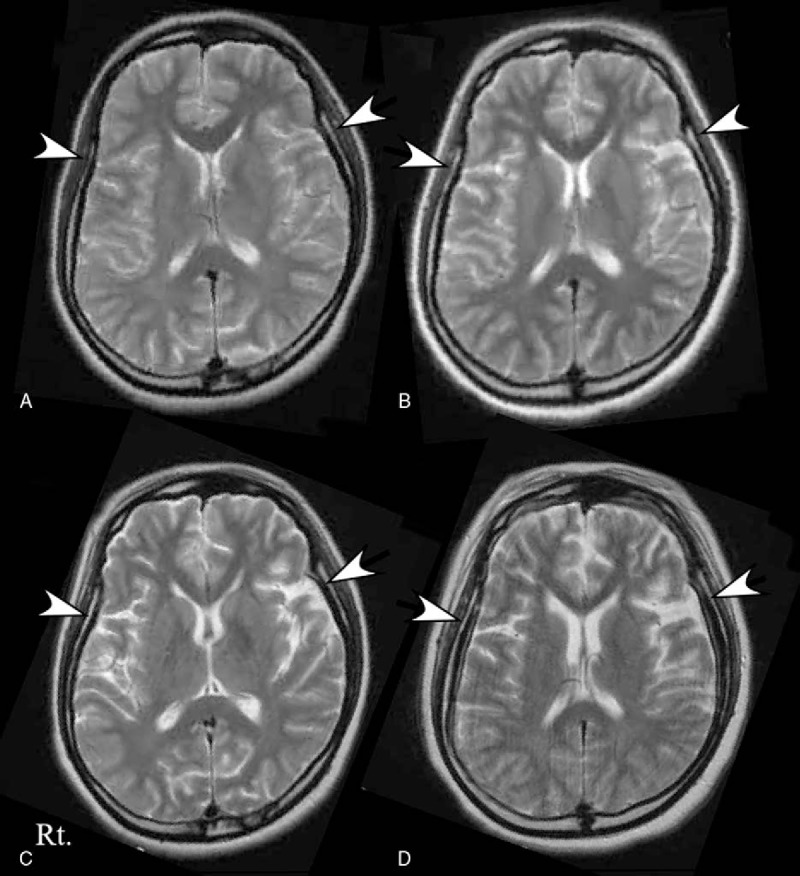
Serial MRI studies in Patient 2. The first brain MRI was normal (panel A). The second brain MRI, obtained on day 19, showed the presence of diffuse brain atrophy (panel B, white arrows). The diffuse brain atrophy showed progression on the third brain MRI, obtained on day 42 (panel C), and the final follow-up MRI, obtained 3 years 8 months after admission (panel D).
